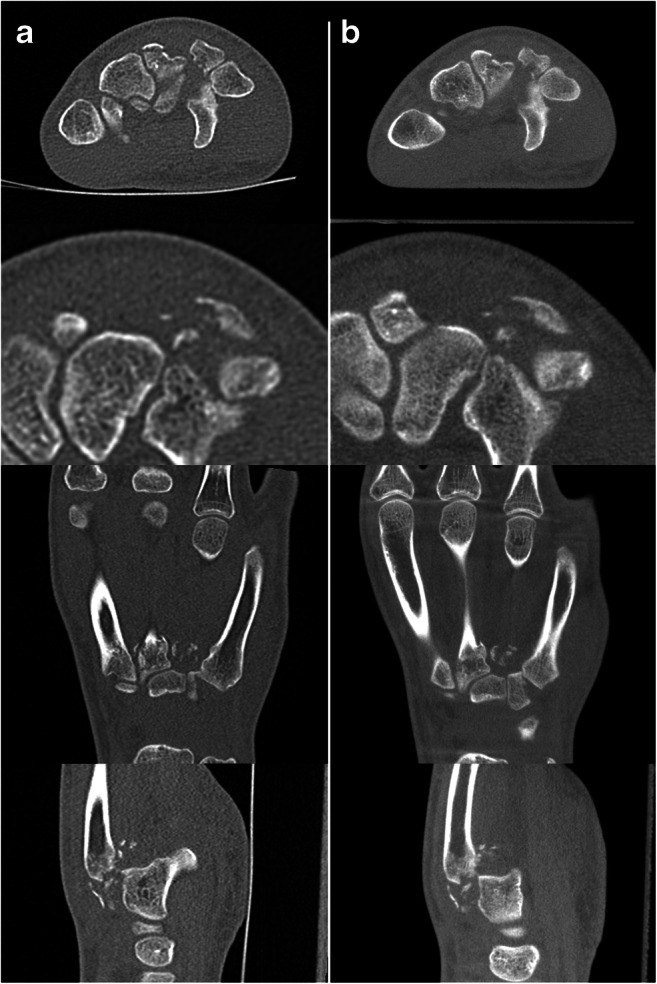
Example of a wrist CBCT (right column) and MDCT (left column) in a 17-year-old adolescent with comminuted fractures of the metacarpal bases and the distal row of carpals. First row axial, second row axial detail, third row coronal, fourth row sagittal reformations, all in 1-mm slice thickness
